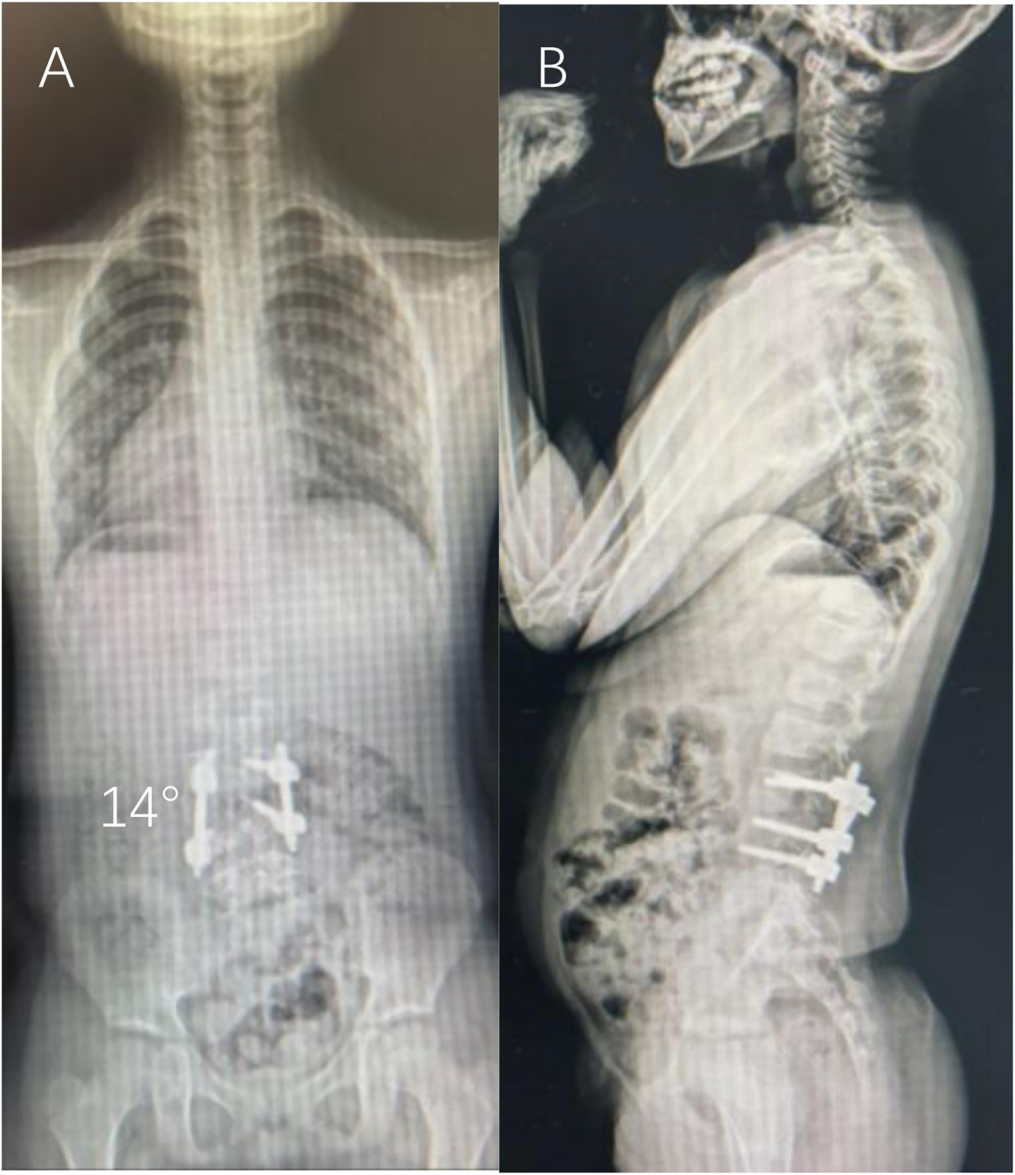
Postoperative full-spine standing radiographs at 6-month follow-up. (A) AP view showing maintained coronal correction with a Cobb angle of 14° and evidence of bony fusion on radiographs at the L4–L5 level. (B) Lateral view confirming the preservation of physiological lumbar lordosis.
